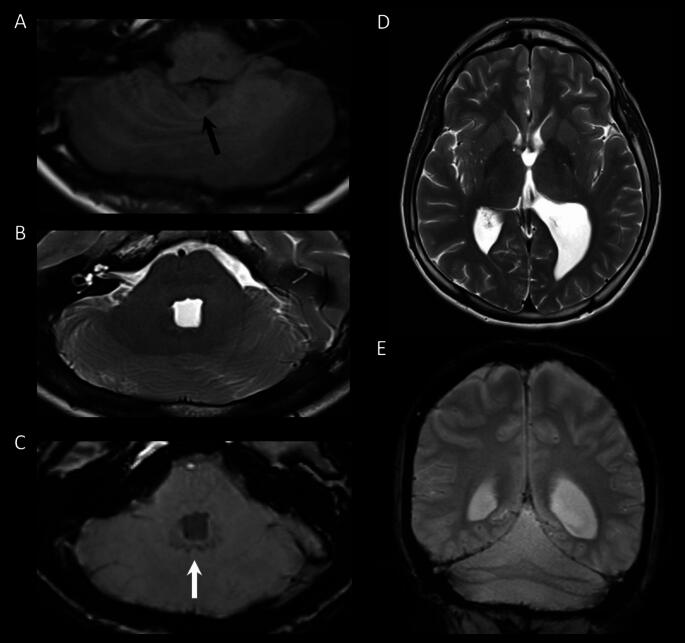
Axial T1-weighted MRI scan at the age of 22 years showing an absent vermis with the nodulus (black arrow) still present (A). T2-weighted axial image showing the fused cerebellar hemispheres, an abnormally square-shaped fourth ventricle, and mild flattening of the ventral pons (B). Axial susceptibility-weighted image depicting an area of hypointense signal crossing the midline dorsal to the fourth ventricle (white arrow) indicating dentate nuclei fusion (C). T2-weighted axial image visualizing the asymmetric enlargement of the left lateral ventricle with dilation especially of the left temporal and occipital horn (D). Coronal multi-echo gradient-recalled echo T2*-weighted image showing dilation of the left occipital horn and fused cerebellar hemispheres (E)
